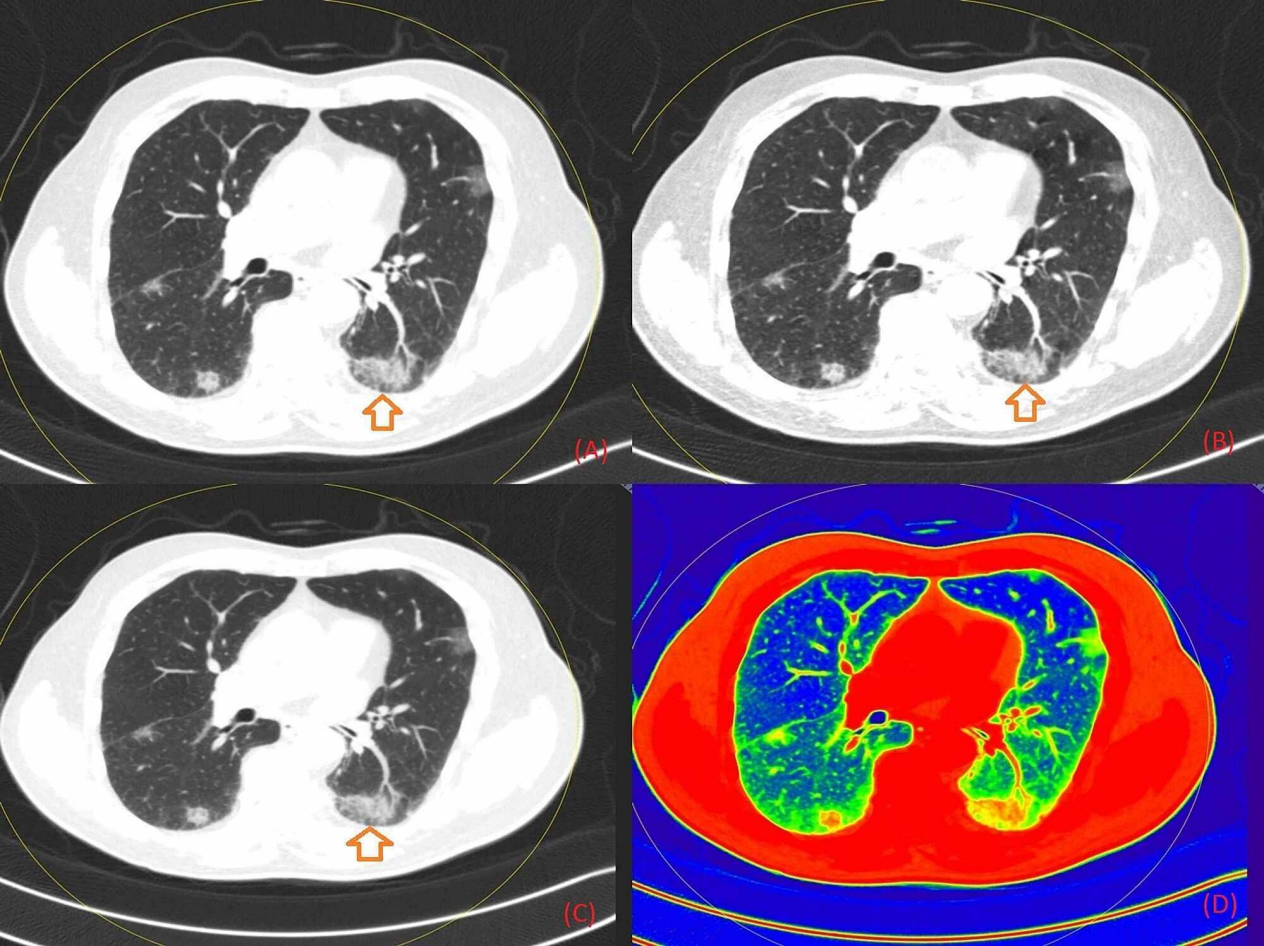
An axial non-contrast chest DECT image obtained in a 42-year-old male shows patchy GGO in the lower basal segment of the right and left (marked with arrows) lung fields Figure 6A is the section in grayscale, Figure 6B in 40 keV energy, Figure 6C in 190 keV energy, and Figure 6D as a color-coded image based on atomic number. DECT: Dual-Energy Computed Tomography; GGO: Ground-Glass Opacity
